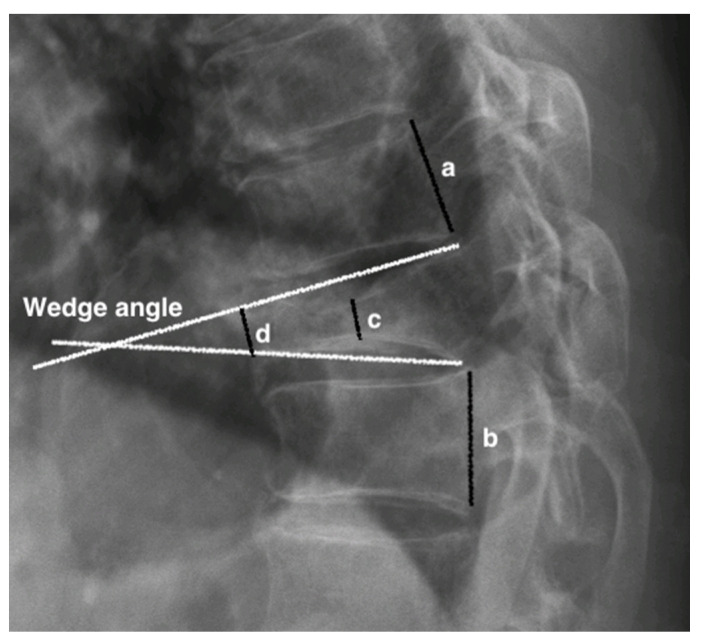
The measurement of radiographic parameters. The wedge angle is defined as the angle between the superior and inferior endplates of the collapsed vertebra. c and d represent the anterior and middle vertebral height, respectively. The normal vertebral height was defined as (a + b)/2. The anterior and middle compression ratios (ACR and MCR) were calculated as [1 − (ant or mid-height/normal height)] × 100%.
